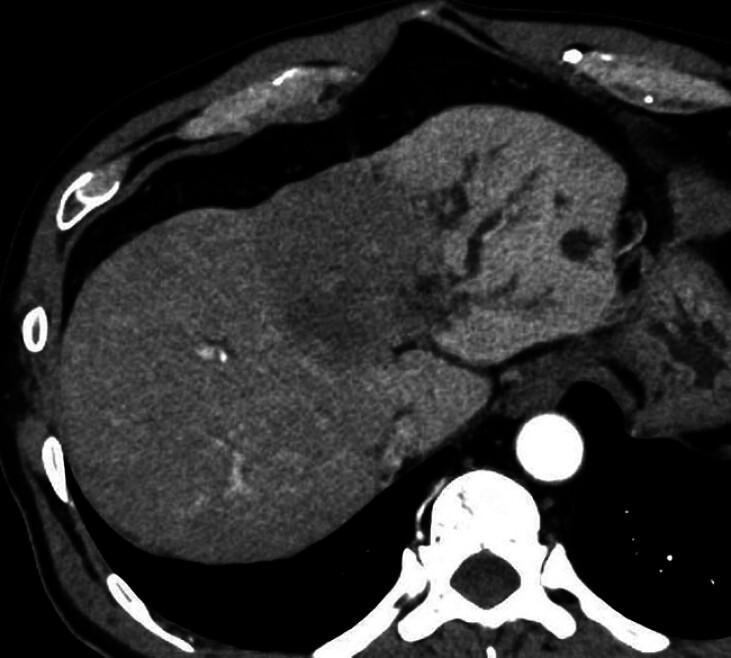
Contrast-enhanced abdominal computed tomography showed a hypovascular tumor in hepatic segment 4.
